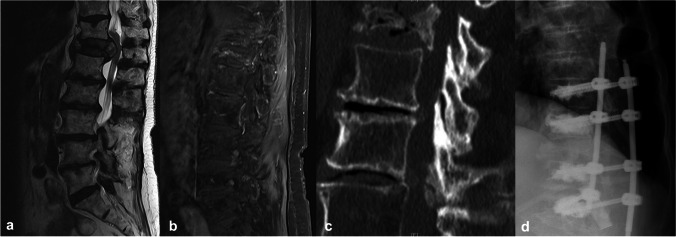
a Sagittal T2-weighted and b postcontrast magnetic imaging of a traumatic Th 12 fracture of an 85-year-old female patient presenting with progressive motor weakness of the low extremities. c Lateral CT scan depicting the osteoporotic Th 12 fracture. d Lateral radiographic view of cement-augmented posterior screw fixation extending from level Th10 to L2
